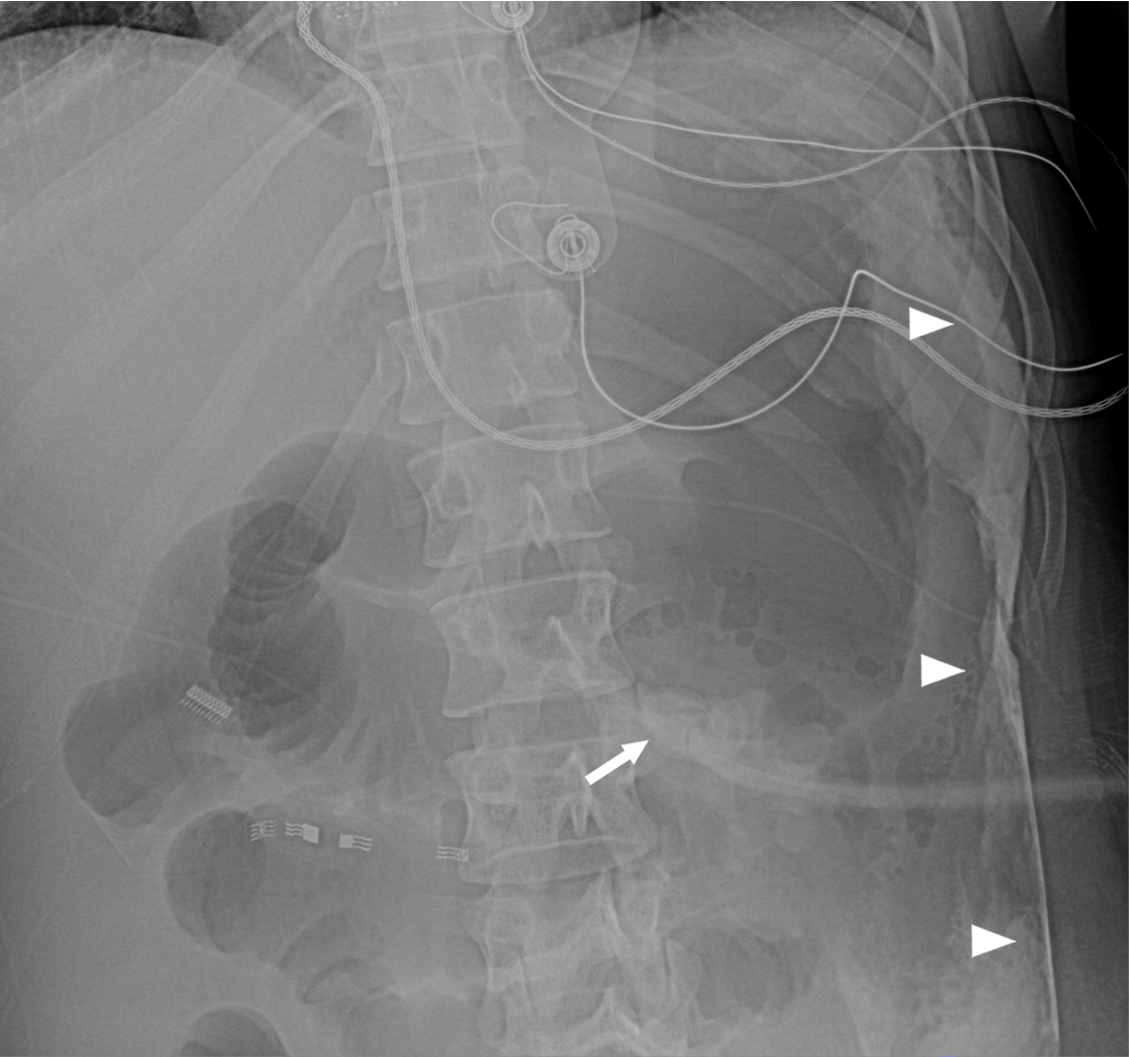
Frontal radiograph with the patient in a right lateral decubitus position shows the G-tube (arrow) overlying the greater curvature of the stomach. Peritoneal contrast leakage (arrowheads) is noted in the left lateral abdomen.
